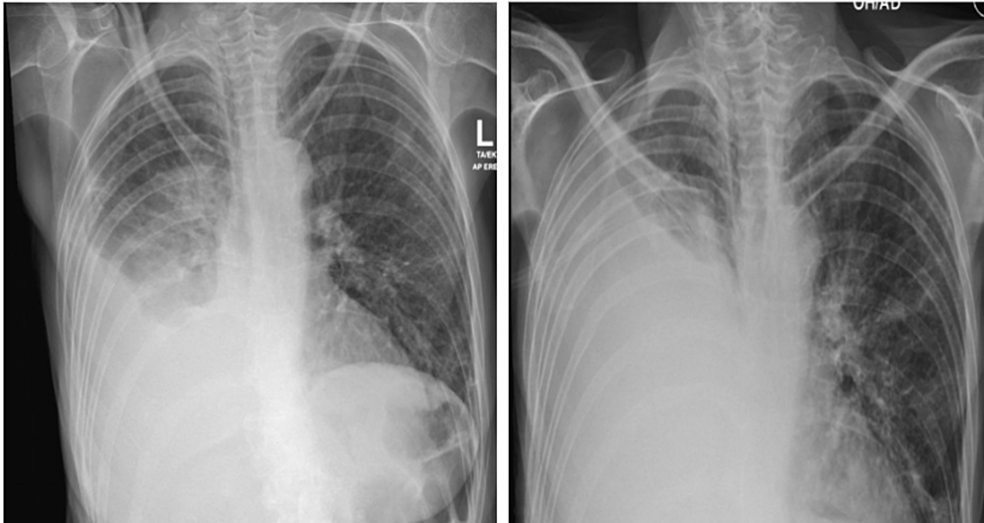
The two chest X-rays performed within a gap of three days reveal worsening right-sided pleural effusion.
